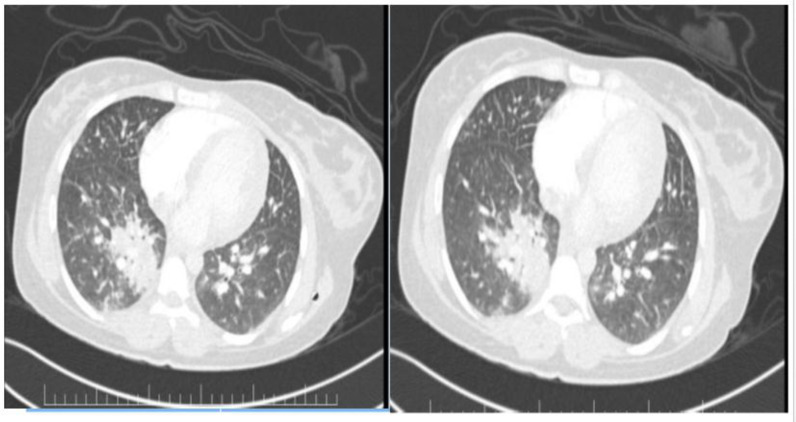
Computed tomography pulmonary angiogram scan – dilated pulmonary trunk, right lower lobe consolidation + associated bilateral diffuse area of ground glass consolidation + few subcentimeter mediastinal lymph nodes and mild pleural effusion + no major pulmonary embolism.
